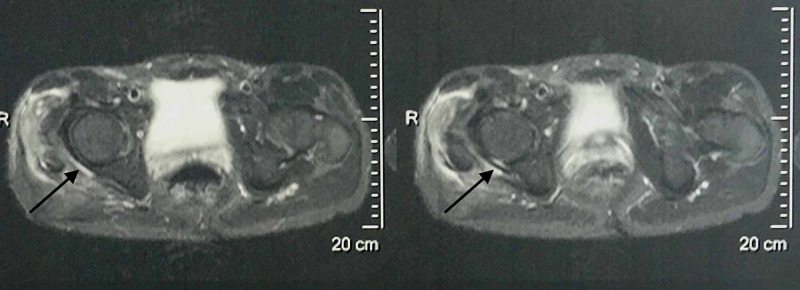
MRI evaluation after medication (transverse plane). The posterior hip capsule signal is improved. The joint space is clear without effusion.
